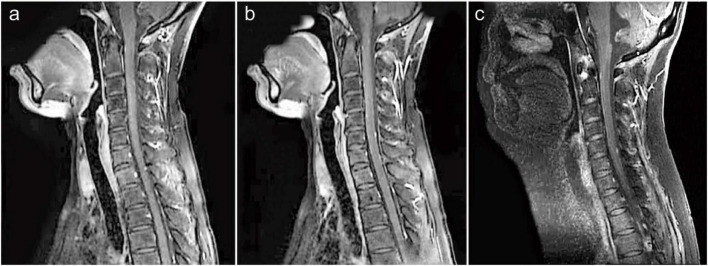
It shows that the lesion in the cervical spinal cord has decreased in size and density. (a,b) Is a magnetic resonance imaging taken in an external hospital in July 2022, and (c) is a magnetic resonance imaging taken in our hospital in October 2022.
